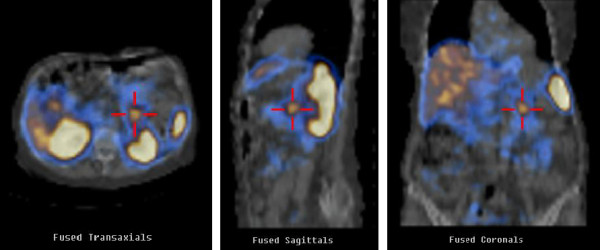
Somatostatin receptor scintigraphy with SPECT acquisition. Area of increased uptake anterior to the left kidney and medial to the spleen, consistent with the pancreatic mass detected by MDTC.
